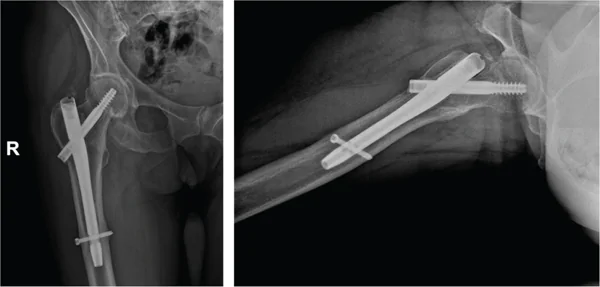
AP and lateral radiographs of right proximal femur after subsequent injury demonstrating right‐sided peri‐implant subcapital neck of femur fracture.
